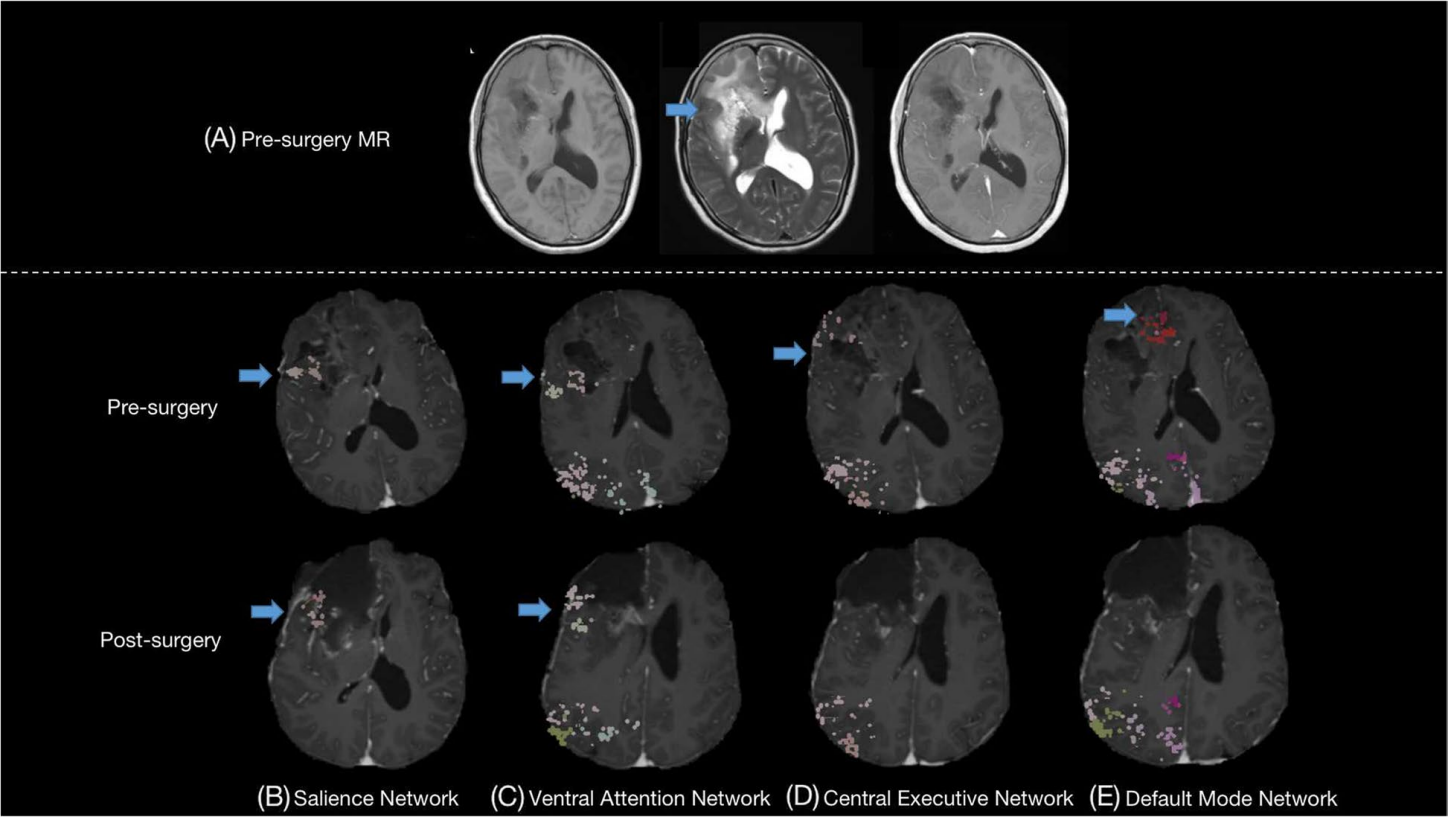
MRI images and the networks showed by the Quicktome in patient no. 2, who had the glioma resection in the right frontal, temporal, insula lobe. A T1, T2, and postcontrast T1 images before surgery; the Quicktome software demonstrates part of the B salience network, C ventral attention network, D central executive network, and E default mode network, where were inside or close to the tumor before surgery and the resection location after surgery
